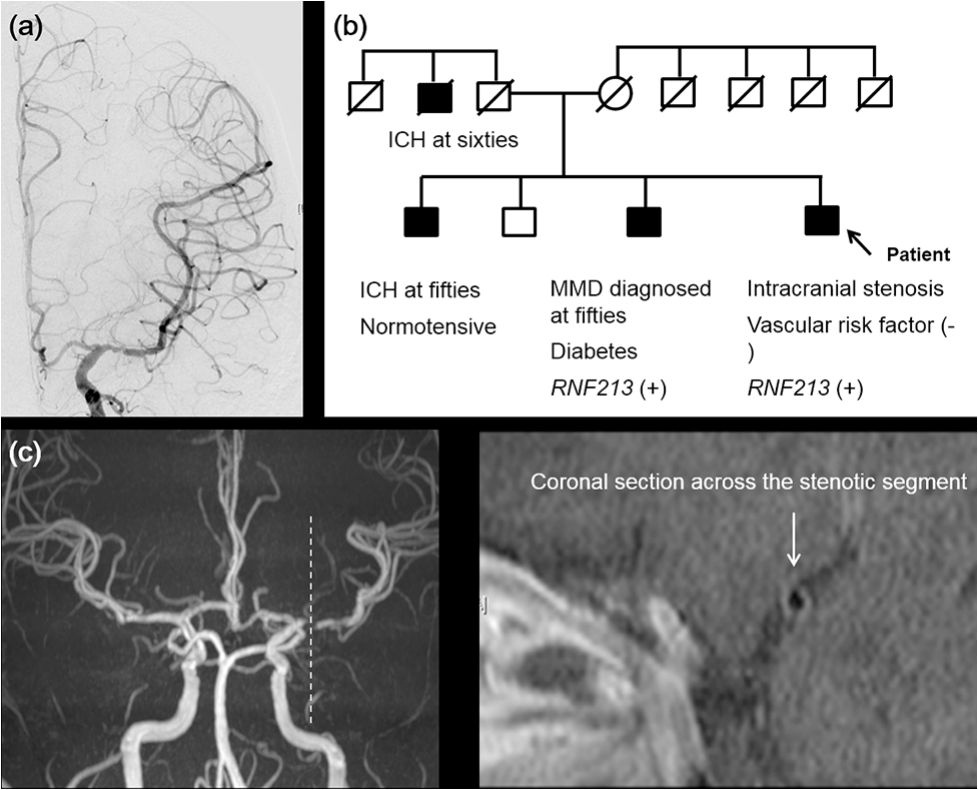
Neuroimaging and genetic findings of a patient with non-Moyamoya-type intracranial arterial occlusive disease. (a) Conventional angiography of a 53-year-old male shows stenosis of the proximal MCA, but intact distal ICA and absence of Moyamoya vessels. (b) Family tree. This patient has a family history of MMD and *RNF213* p.Arg4810Lys mutations. Small black points indicate members who were directly examined. (c) High-resolution MRI reveals a smaller outer diameter (2.32 mm) and the absence of focal plaque in the stenotic segment (arrow). ICH, intracranial hemorrhage.
